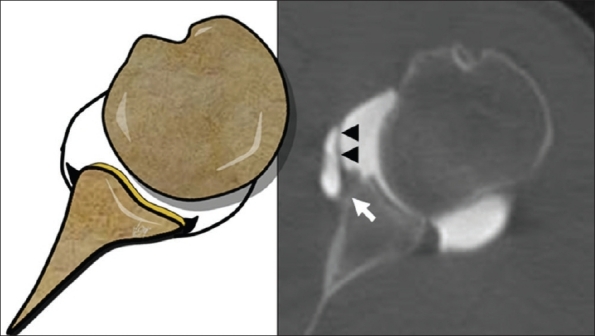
Axial CT-Arthrography image of a 25-year-old man with arthroscopy proved ALPSA lesion. The anterior labrum is displaced medially (*arrow*) and the capsule is intact (*arrowed*)
